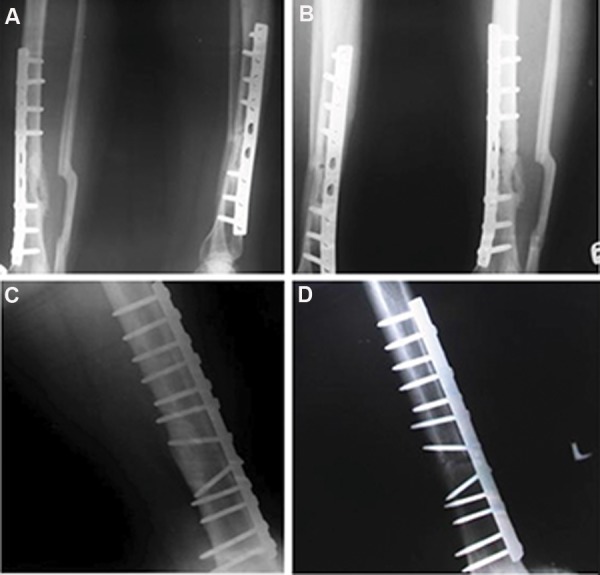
Patients radiographies. A. Anteroposterior and lateral radiographs of a non-united open tibial and fibular fracture of a 32-year-old
